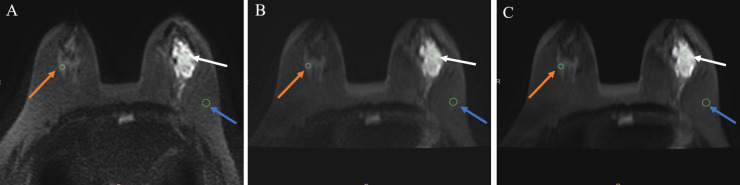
A 51-year-old female with breast cancer in the left breast. A, conventional DWI; B, FOCUS DWI; C, FOCUS-DLR DWI. This image shows the delineation of the ROIs, where the white arrow indicates the lesion ROI, the blue arrow indicates the background ROI, and the orange arrow indicates the glandular tissue ROI. DLR, deep-learning-based reconstruction; DWI, diffusion-weighted imaging; FOCUS, field-of-view optimized and constrained undistorted single-shot; ROI, region-of-interest.
